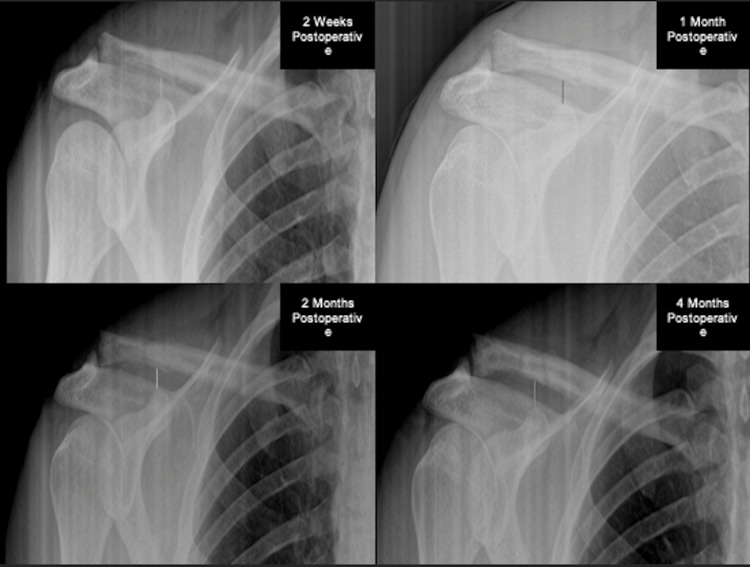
AP radiographs of subject 3 with two weeks, one month, two months, and four months postoperative radiographs available. At the subject’s two weeks visit, the CC distance is 7.8mm. During the one-month visit, the CC distance is 8.0mm. At the two months visit, CC distance is 8.1mm. At the four months visit, the CC distance slightly increased to 10.3mm. Image credit: Alvarho Guzman
